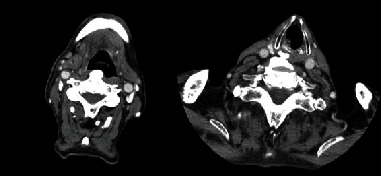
The axial CT scan of the patient's neck.
